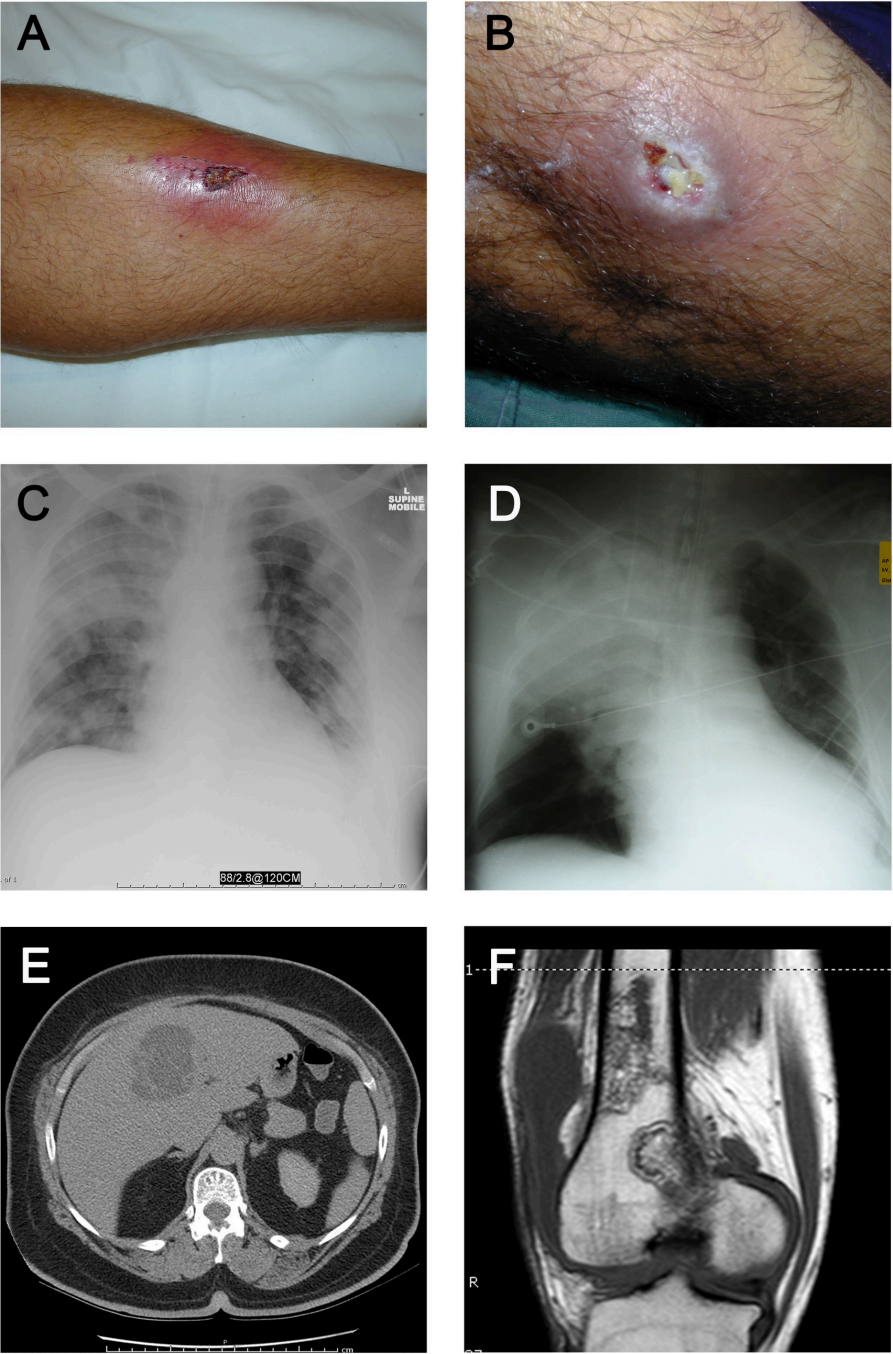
Clinical and radiologic images of melioidosis cases in the Katherine region. (A) Cutaneous melioidosis; (B) Cutaneous melioidosis; (C) Non-fatal bacteremic melioidosis pneumonia; (D) Fatal bacteremic melioidosis pneumonia; (E) Computed tomography scan demonstrating a liver abscess; (F) Magnetic resonance imaging scan demonstrating osteomyelitis of the femur. Photos taken by Prof Bart Currie.
